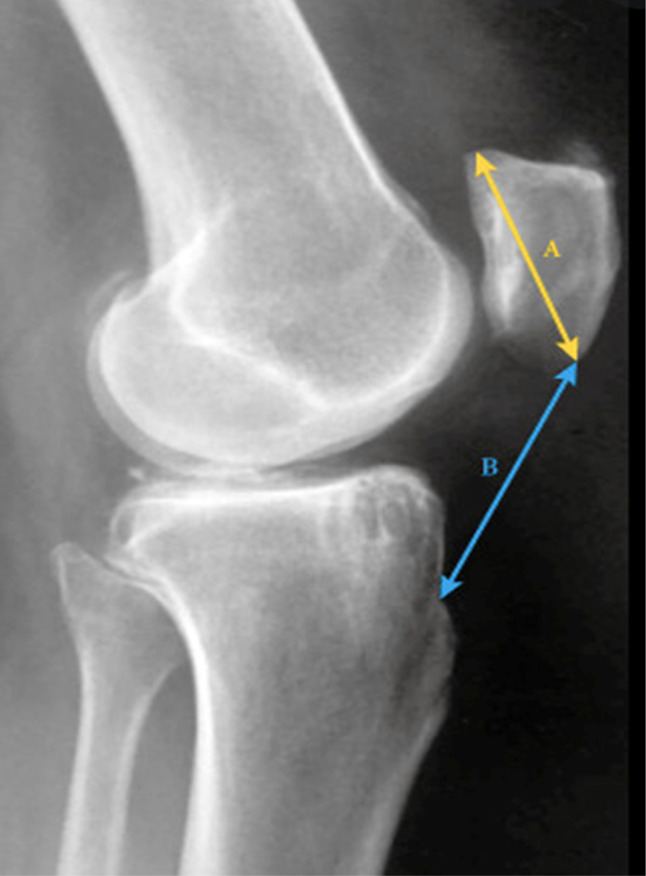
Radiographic determination of ISI. Defined as the PTL over the PL. “A” represents the PL, which is the longest distance from the superior pole of the patella to the inferior most aspect of the patella. “B” represents the PTL, which is the distance from the inferior most aspect of the patella to the superior aspect of the tibial tubercle. ISI = Insall-Salvati Index, PL = patellar length, PTL = patellar tendon length
